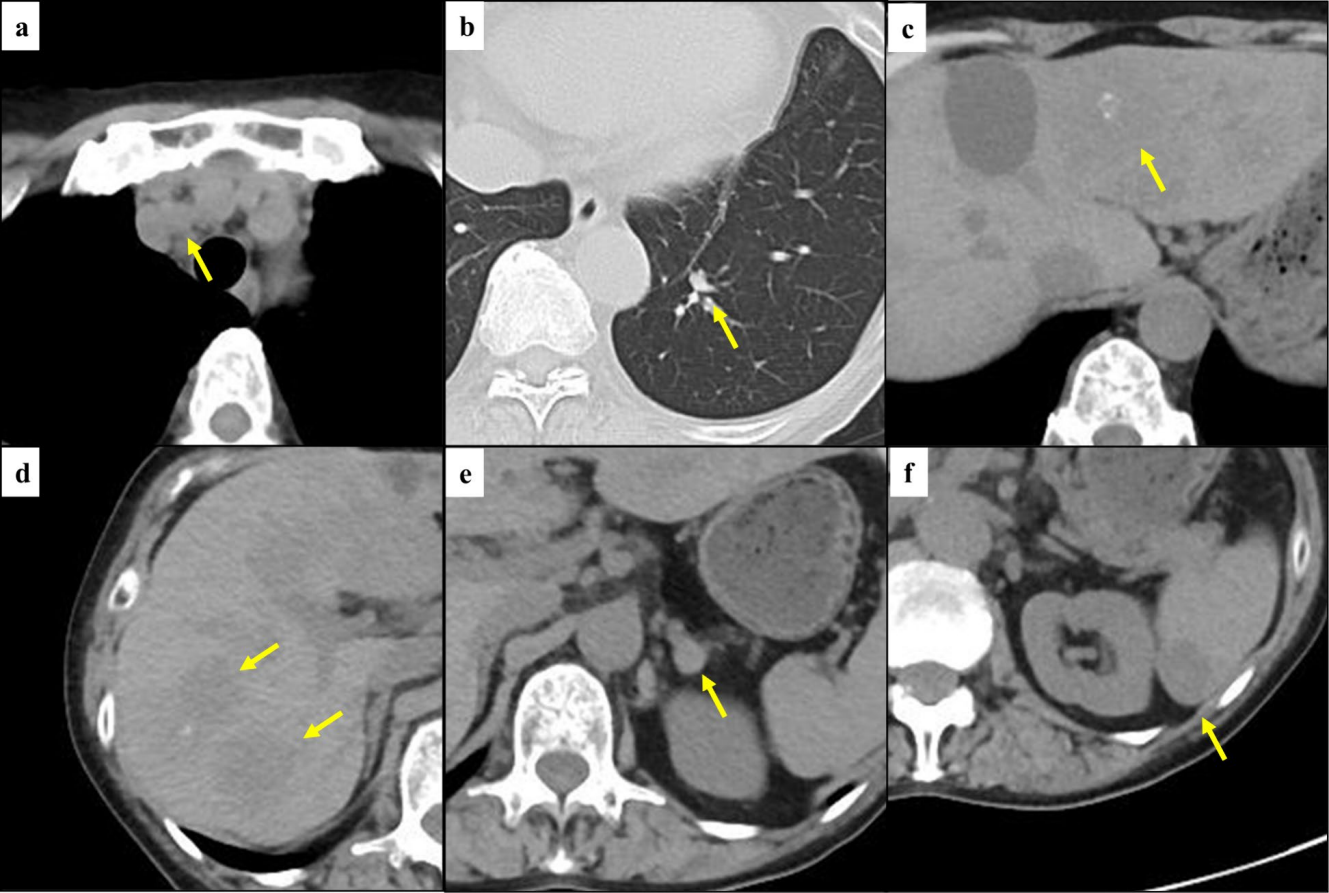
Computed tomography before larotrectinib initiation. The computed tomography before larotrectinib initiation showed mediastinal lymph node (a), lung (b), liver (c, d), left adrenal gland (e), and splenic (f) metastases
